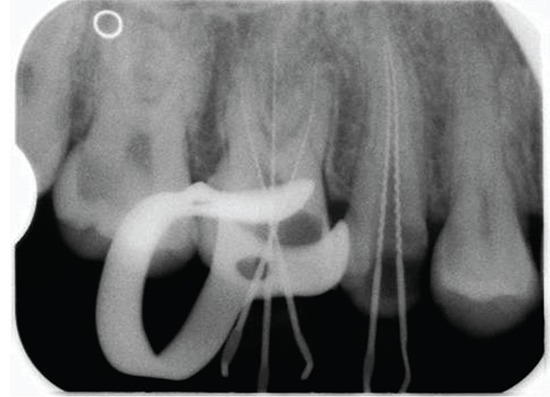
Endodontic files within 2 mm of the radiographic apex of teeth.
